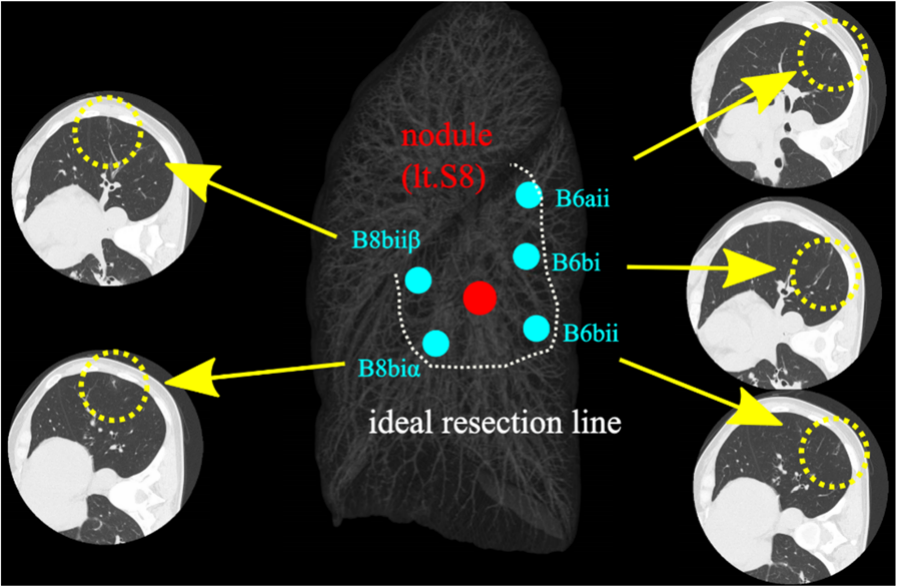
Three-dimensional configuration of the CT for “mapping” for the second operation
